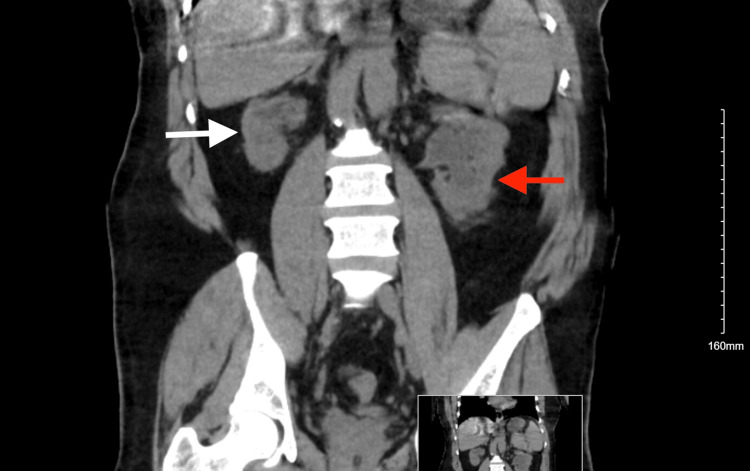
CT of the abdomen and pelvis (coronal view) without contrast showing atrophic right kidney (white arrow) and left hydronephrosis (red arrow) with bilateral cortical scarring CT: computed tomography
